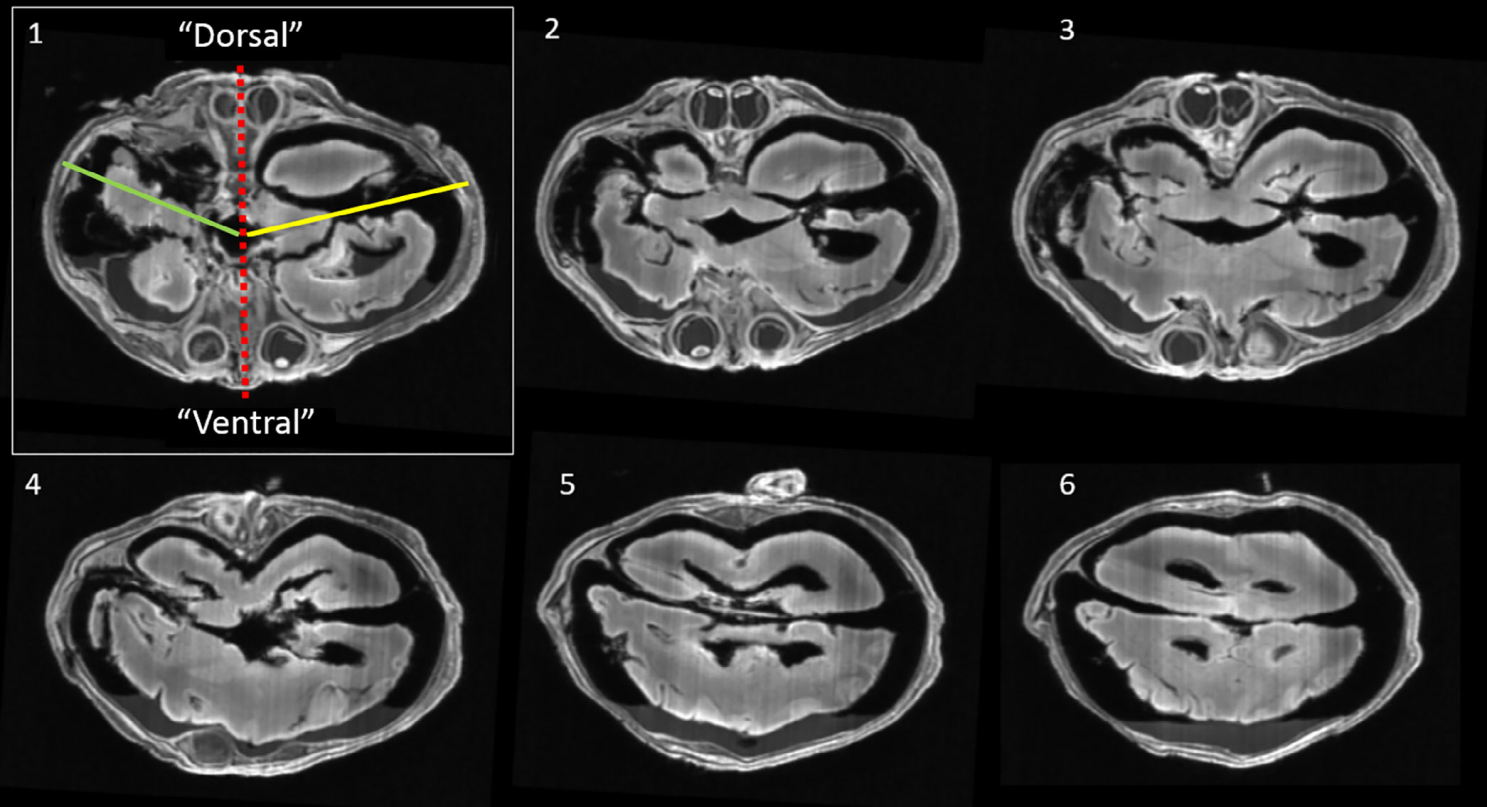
Transverse T1‐weighted magnetic resonance images of the cranial region of the laterally‐deviated cephalothoracoileopagus depicted in Figure 9. Image numbers indicate caudal (1) to cranial (6) positions respectively. Image 1 is accompanied by a red dotted anterior to posterior axis, indicating the midline location of both the divided and diverted facial primordia of each twin, hence dividing the “right“ from the “left” twin. The yellow line indicates the midline of the “left” divided and diverted brain, the green line that of the “right” divided and diverted brain. Clearly noticeable in image 1 is that the smallest angle between the two brains is oriented to the holoprosencephalic or “posterior” face. Note that the frontal lobes, anterior horns of the lateral ventricles and the third ventricles of each twin are conjoined. Unfortunately, the brain is somewhat macerated, potentially interfering with its interpretation. However, and interestingly, in contrast to the facial appearance, the compound brain does not in our opinion show the typical aspects of holoprosencephaly
